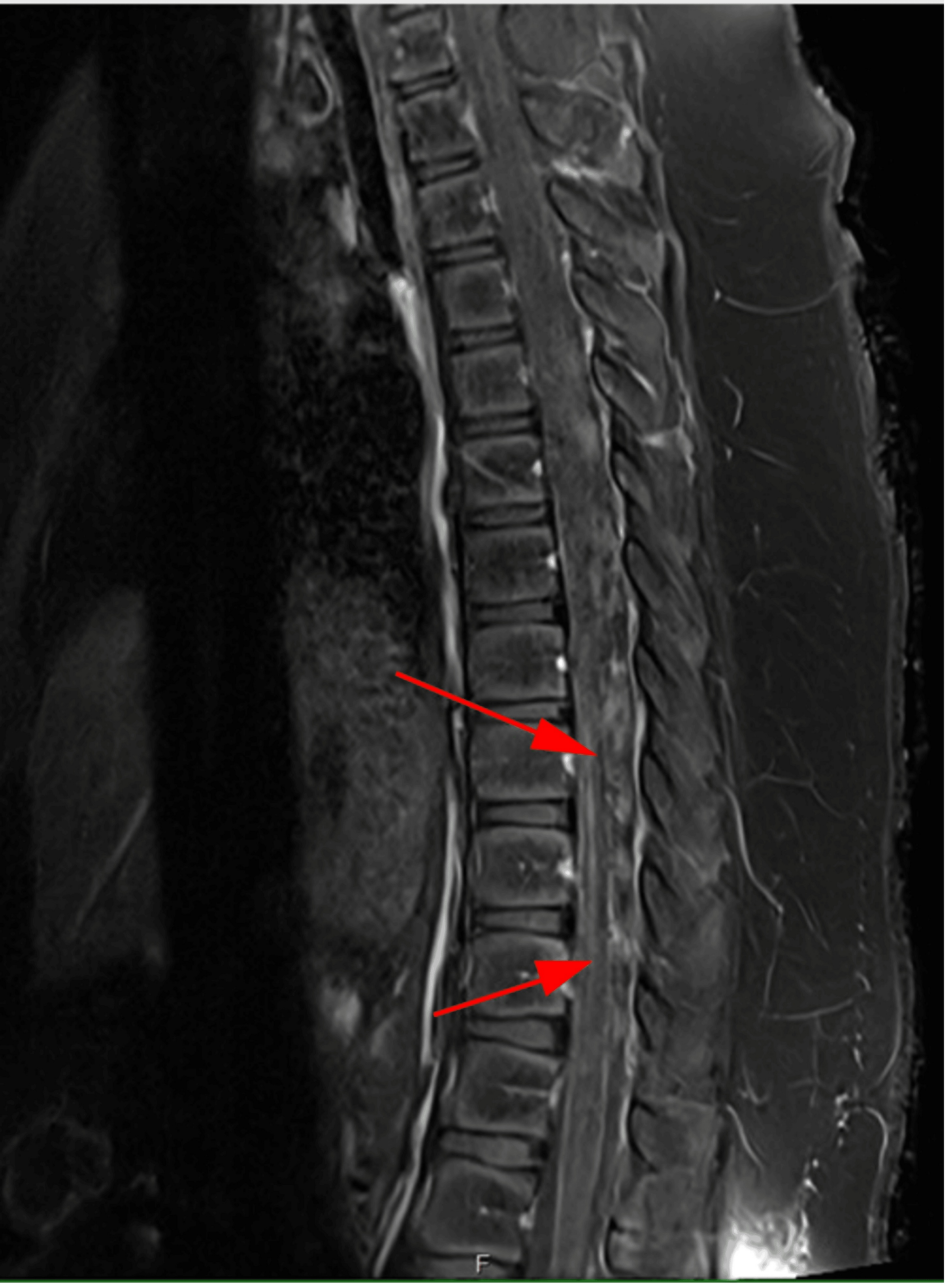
Sagittal post-contrast T1-weighted MRI of the thoracic spine showing no evidence of enhancement from T8 to T9 through T11 to T12, indicated by red arrows
